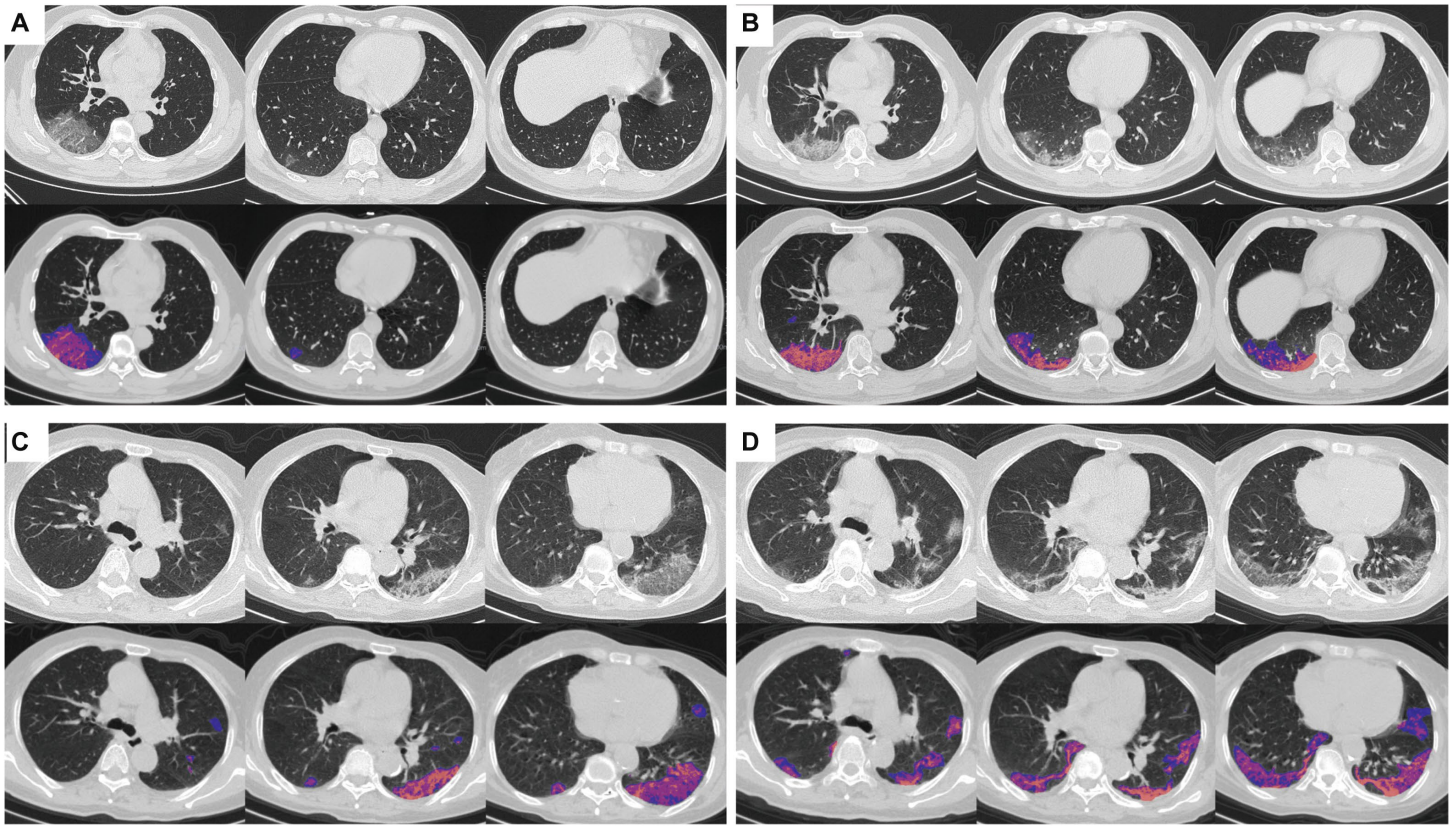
COVID-19 pneumonia lesions detected by the AI system and visualized as pseudo colors. First rows: initial CT images; second rows: AI auto-segmentation displayed with blue pink, and red pseudo colors representing ground-glass opacity (GGO), semi-consolidation and consolidation, respectively. (A,B) First CT imaging (A) and fellow-up after 3 days (B) of a 56-year-old male patient, who did not meet the endpoint during the follow-up. (C,D) First CT imaging (C) and fellow-up after 4 days (D) of a 80-year-old female patient, who reached the endpoint of progression to severe illness and died after 13 days.
